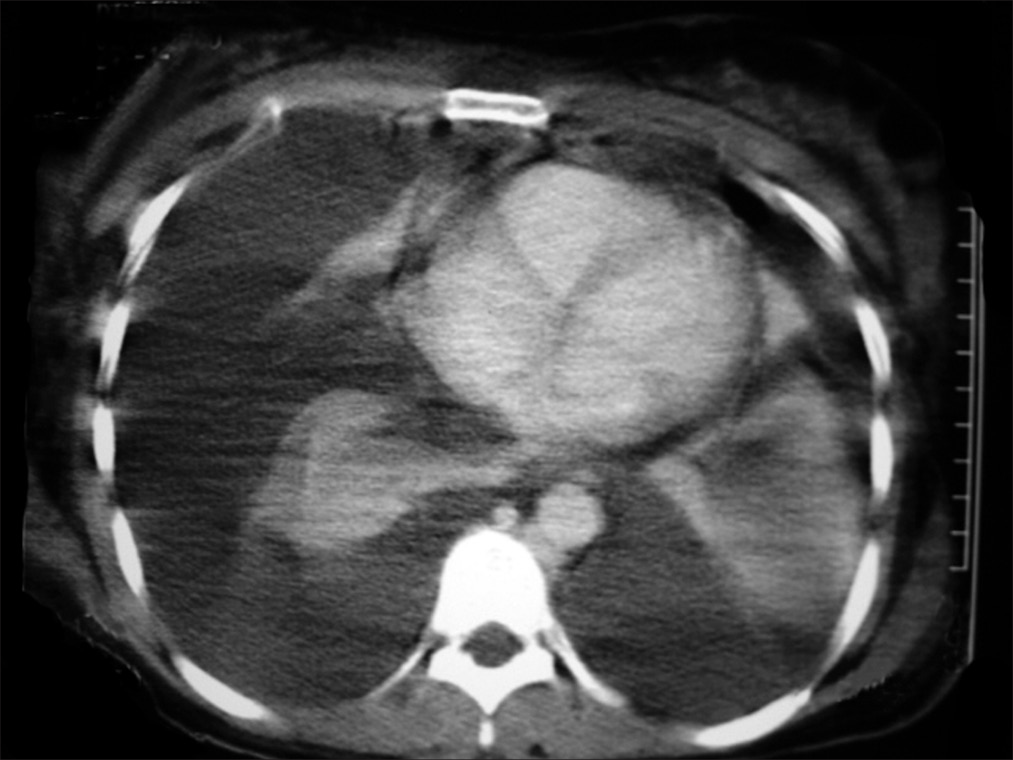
CT of thorax showing bilateral pleural and pericardial effusion
